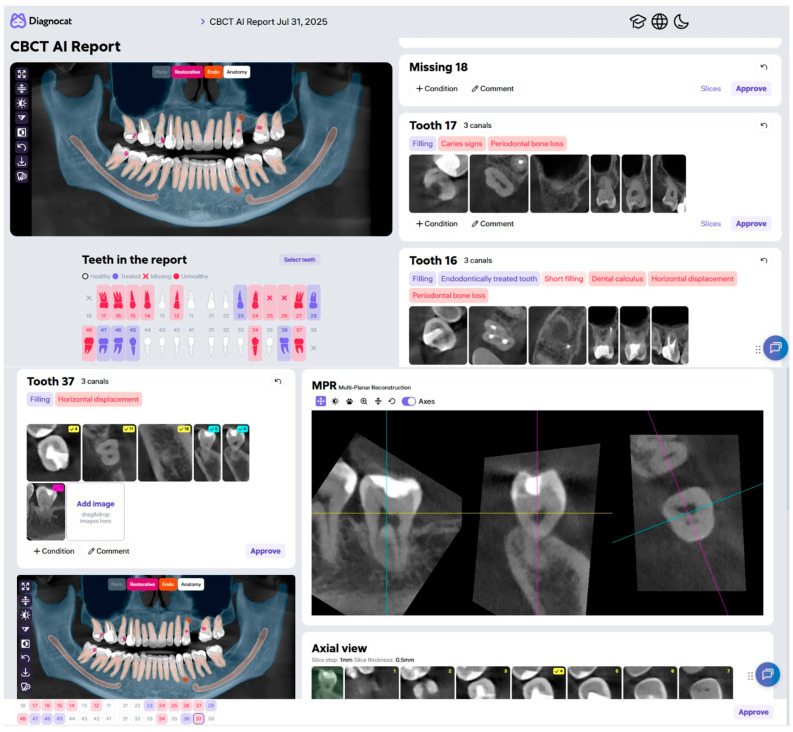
Screenshot of the AI Program “Diagnocat”—Report from CBCT analysis.
